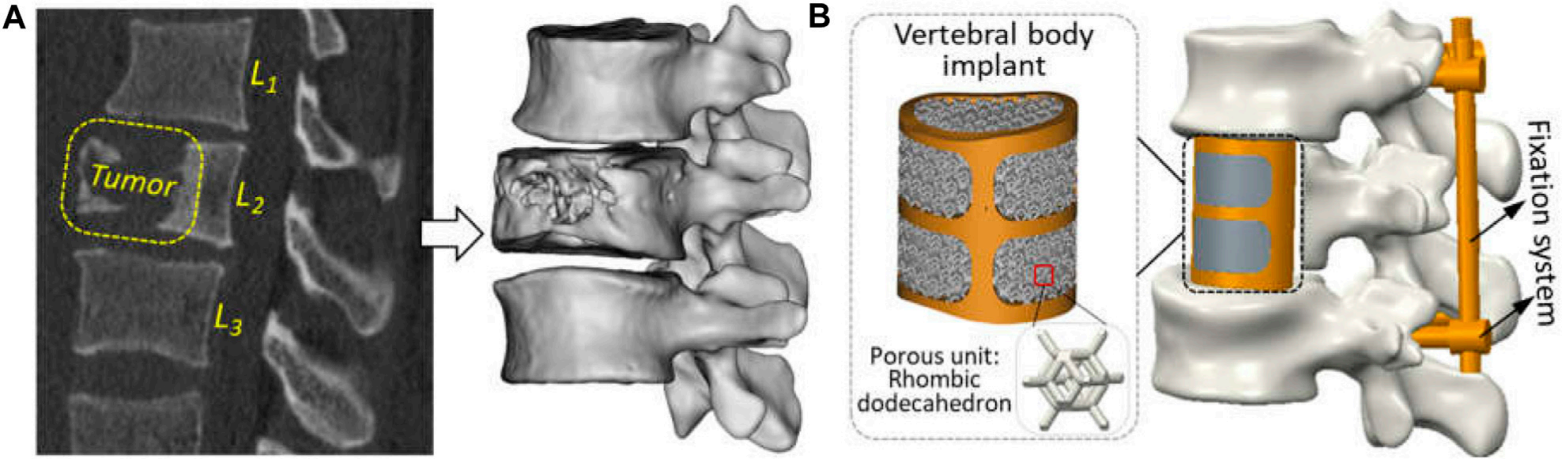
Reconstruction of geometric models for clinical treatment of vertebral defect: (A) tumor observation in the CT scanning image and three-dimensional geometric modeling; (B) vertebral replacement using by the artificial vertebral body implant and posterior fixation system.
